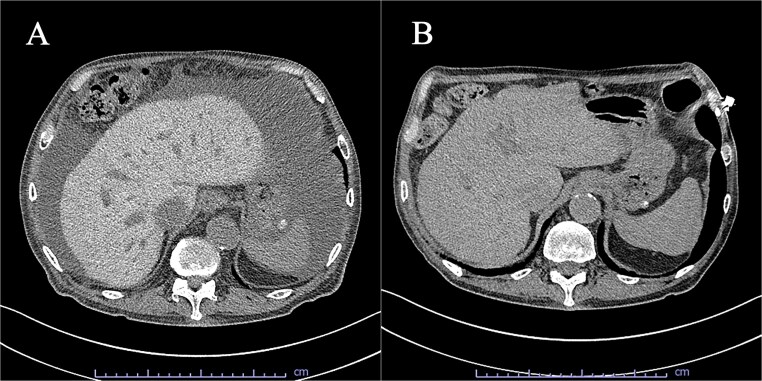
Abdominal computed tomography showing (A) increased hepatic attenuation and ascites and (B) resolution of ascites after discontinuation of amiodarone.
